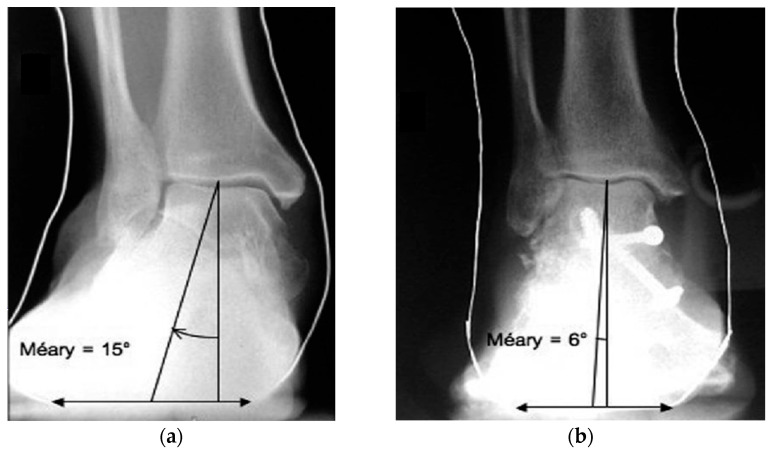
Hindfoot angle (Méary) measured on frontal weight-bearing radiographs with hindfoot alignment view. Preoperative view (a) and postoperative view at final follow-up (b).
